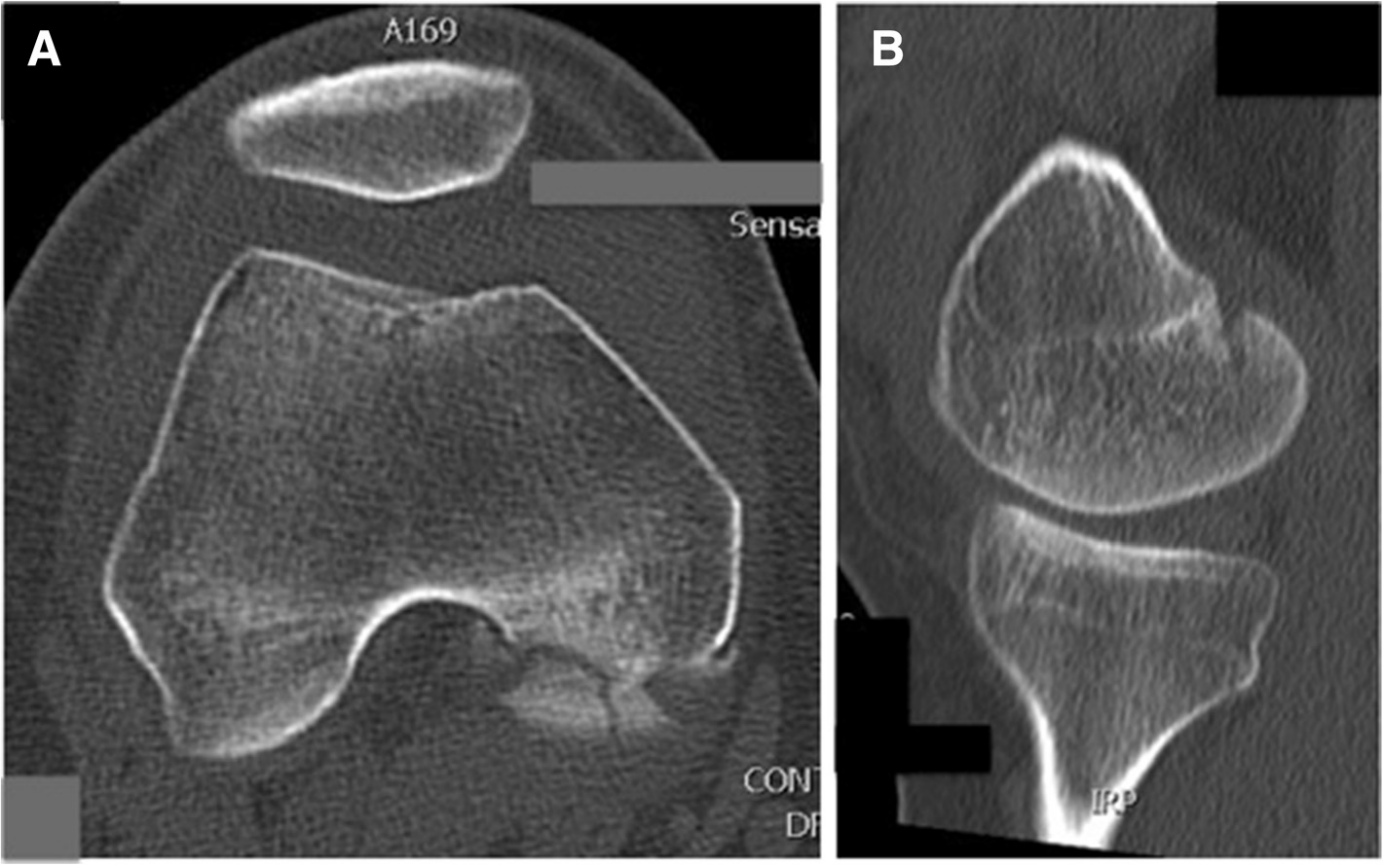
CT-scans provide additional information concerning articular involvement. (A) Coronal image of a Hoffa's fracture. (B) CT reconstruction of a Hoffa's fracture.
